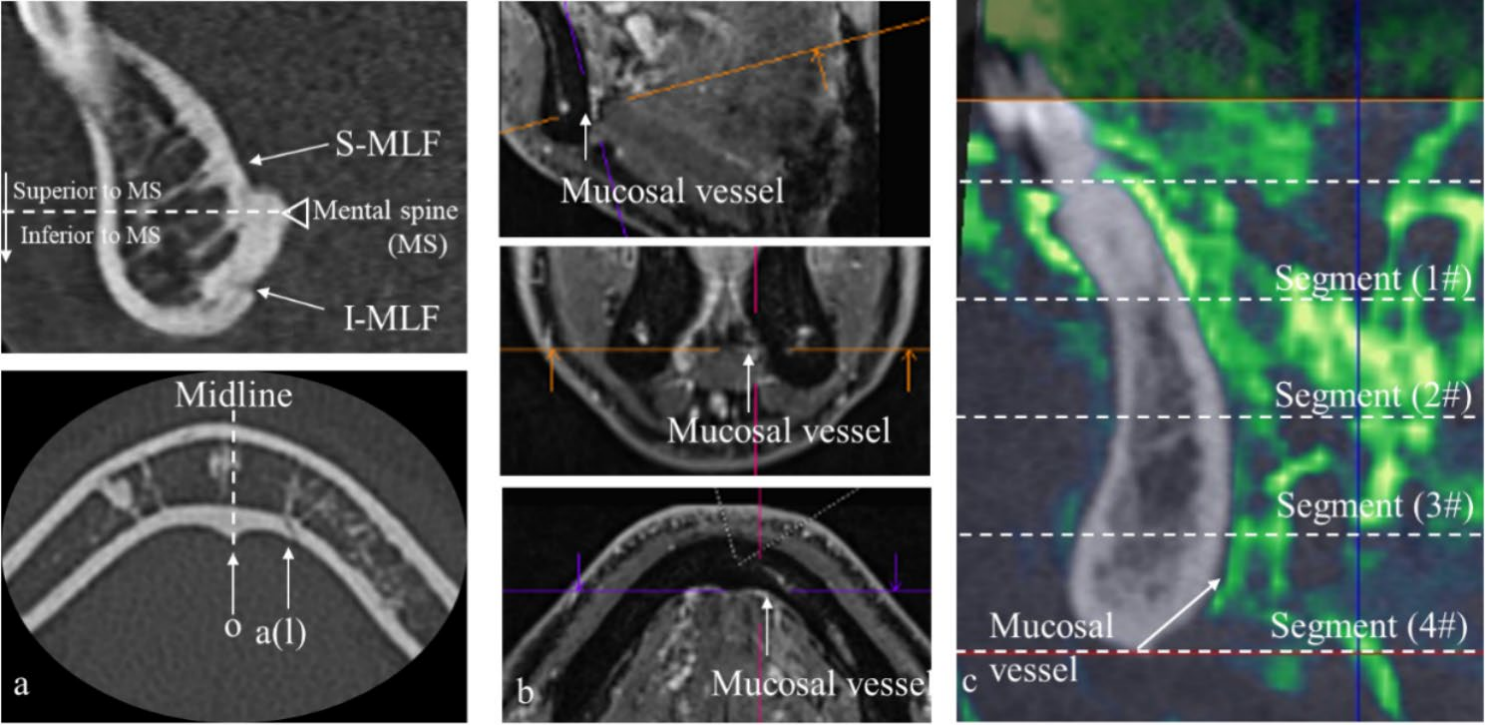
Evaluation of MLFs and LMVs a. Position of MLFs in relation to mental spine in CT (sagittal image, horizontal image respectively). MLFs: mandibular lingual foramens; S-MLF: superior to the mental spine on the midline; I-MLF: inferior to the mental spine on the midline; a(l): left side to the midline; o: on the mental spine b. LMVs in MRI (sagittal image, coronal image, horizontal image respectively).LMVs: lingual mucosal vessels. The lingual and facial vessels were searched in thier anatomic location firstly and then sublingual and submental vessels. When the high signal intensity of these veseels extended the surface of the mandibular lingual cortex, they were marked it as LMVs c. CT/MRI fusion volumetric image of mucosal vessels and tooth position (sagittal image). 4 average segments were divided from the alveolar bridge to the lowest point of the mandibular inferior border # refers to the segment of tooth
